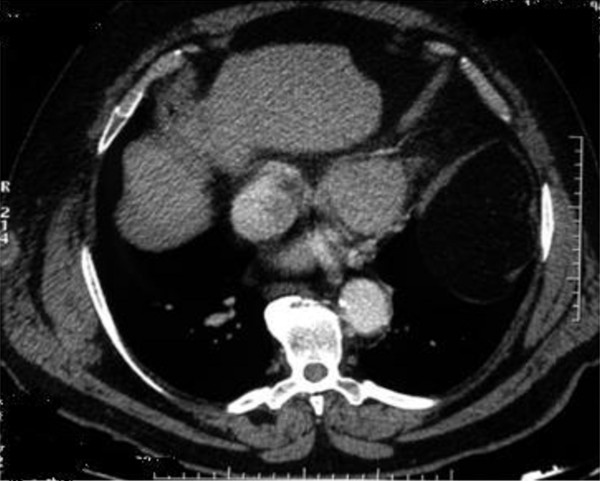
CT scan showing a contrast enhanced hypodermic 38 × 22 mm nodular lesion on the right chest.
